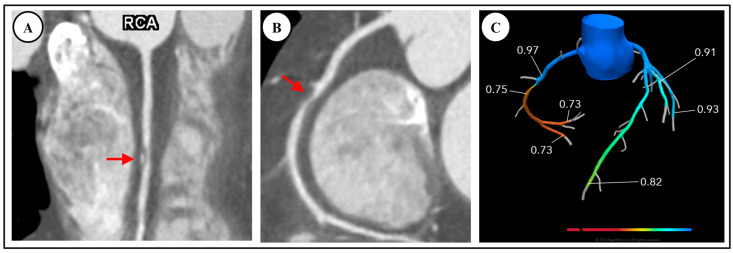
A 59-year-old male reviewed in the outpatient clinic with stable angina and multiple cardiovascular risk factors, found to have moderate CAD with ischemia. (A,B) Linear and curved multiplanar reconstructions on CCTA respectively demonstrate moderate stenosis in right coronary artery (RCA), red arrows, reported as 50–70% (CAD-RADS = 3). (C) FFR-CT positive for ischemia and suggesting obstructive coronary artery disease in right coronary artery. Patient was referred for invasive coronary angiography.
